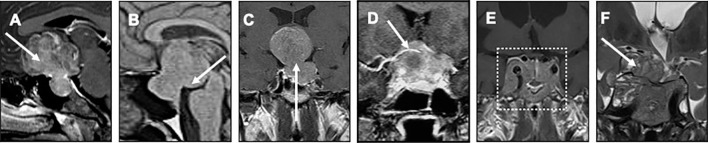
Different patterns of giant tumors extension Preoperative pituitary MRI are presented, including gadolinium-enhanced T1 weighted sagittal (A, B), coronal (C–E) and T2 weighted coronal (F) images. (A) subfrontal extension (white arrow); (B) posterior fossa extension (white arrow); (C) suprasellar extension with a “narrow neck” aspect between the intrasellar and the suprasellar tumor components; (D) encasement of the right anterior cerebral artery (white arrow); (E) massive invasive sphenoid tumor bridging the two cavernous sinuses, defined as “sphenoid arch” aspect (white dotted rectangle); (F) tumor extension through the roof-top of the right cavernous sinus (white arrow).
